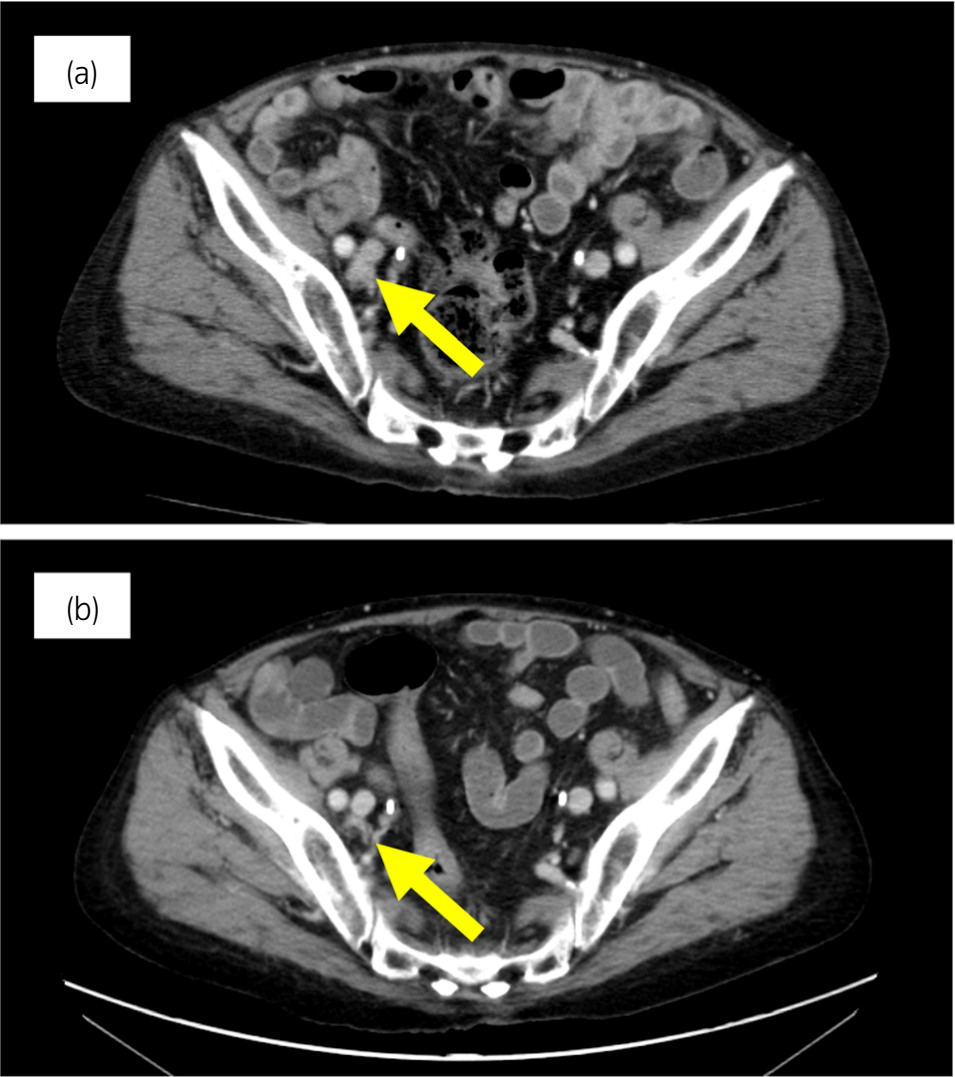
Case 2: CT images of the pelvic lymph nodes before olaparib treatment and 3 months after treatment. (a) Before treatment and (b) 3 months after olaparib treatment. Yellow arrow shows metastatic lymph node.
